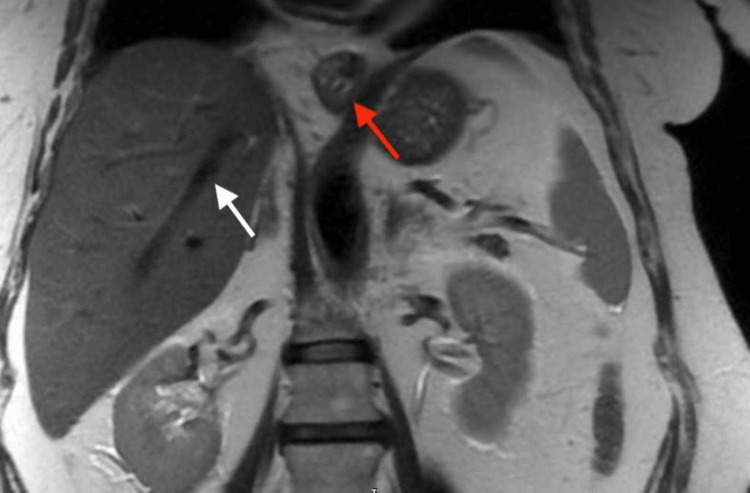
MRI with a contrast agent Transhiatal gastric hernia (red arrow) without intrahepatic and extrahepatic bile duct dilations and with a lumen of normal thickness (white arrow).
